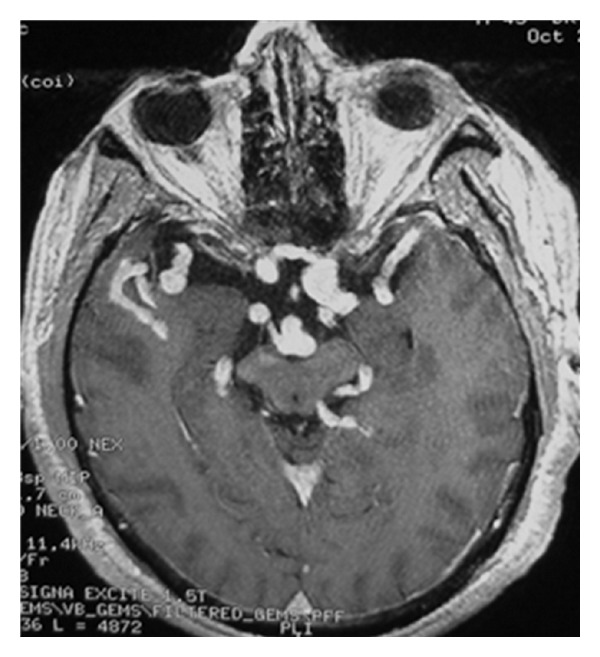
Cerebral MRI showed persistent multiple aneurysms 6 months after treatment.
